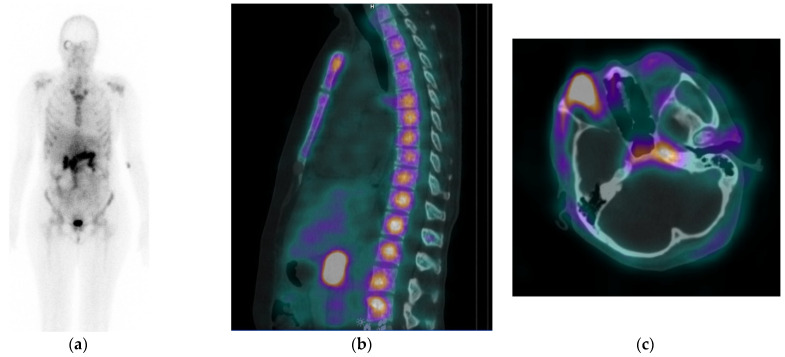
(a) Whole body scan of Patient 1, obtained 3 h after injection of [^99m^Tc]Tc-DB15 in the anterior projection showing physiological accumulation in the pancreas and increased uptake in the skeleton. (b) SPECT/CT sagittal image obtained 3.5 h after injection of [^99m^Tc]Tc-DB15 showing increased accumulation in the vertebrae. (c) SPECT/CT transaxial image showing elevated accumulation in the right orbital wall and in the sphenoid bone.
